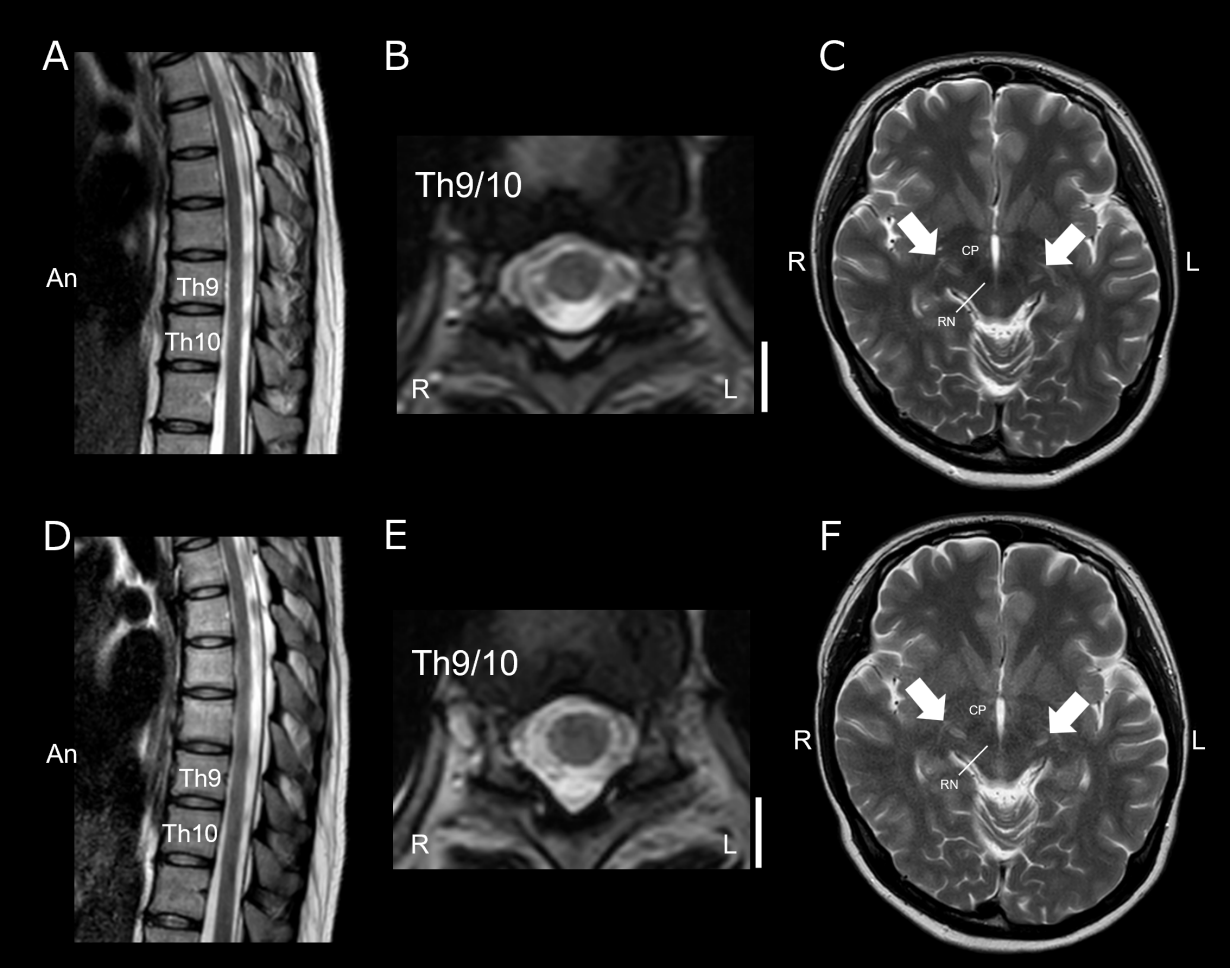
Spinal cord and brain MRI images at the initial hospitalization and one month later. At the initial hospitalization, sagittal T2-weighted images (T2WI) of the thoracic spinal cord (A) showed no abnormal lesions. Although clinical findings suggested lesions around the Th9/10 level, axial T2WI showed no intramedullary abnormal signals (B) Brain MRI revealed mild hyperintense changes in the bilateral pyramidal tracts on T2WI (C, white arrows). Following intravenous methylprednisolone pulse therapy, spinal cord MRI one month later also showed no intramedullary abnormal signals [(D) sagittal T2WI; (E) axial T2WI at the Th9/10 level]. Brain MRI showing mild hyperintense changes in the bilateral pyramidal tracts on T2WI, similar to the initial findings (F, white arrows). White bars correspond to 10 mm. R, right; L, left; An, anterior side; CP, Cerebral peduncle; RN, Red nucleus.
